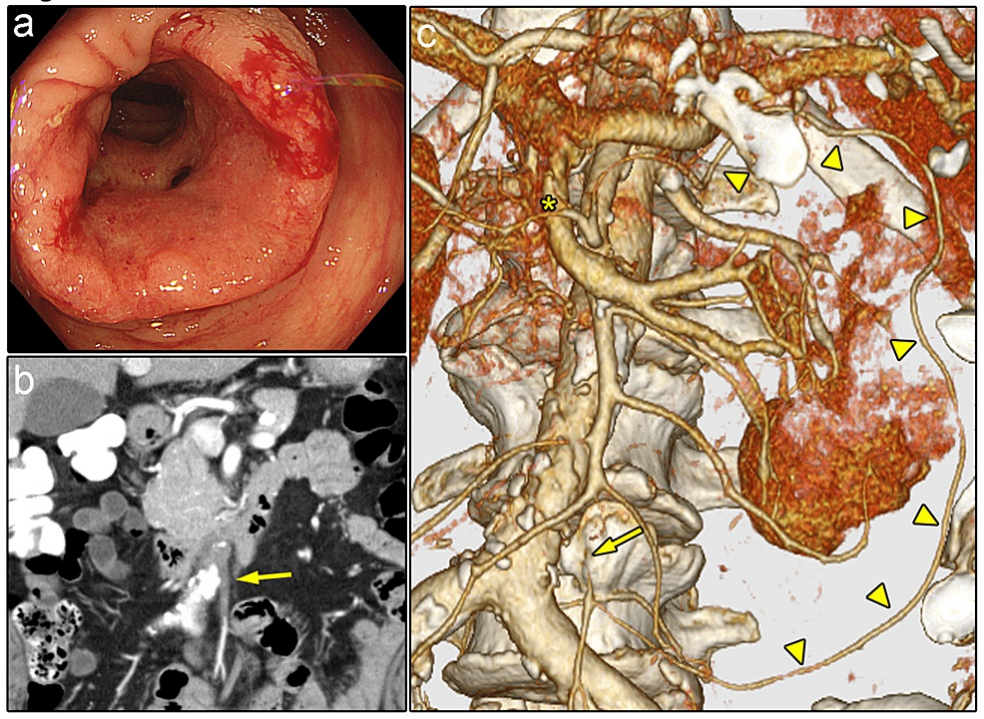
Preoperative images for case two a) Colonoscopy revealed the growth of an advanced tumor. b) CT showed that the root of the IMA was occluded (arrow). The SRA was patent. c) CT angiography demonstrated no blood flow at the root of the IMA (arrow). The distal branches were compensated. The LCA formed an arterial arcade with the AMCA (arrowheads), which shared the same origin as the MCA in this case. The asterisk indicates the left and right branches of the middle colic artery. AMCA: accessory middle colic artery; CT: computed tomography; IMA: inferior mesenteric artery; LCA: left colic artery; MCA: middle colic artery; SRA: superior rectal artery
